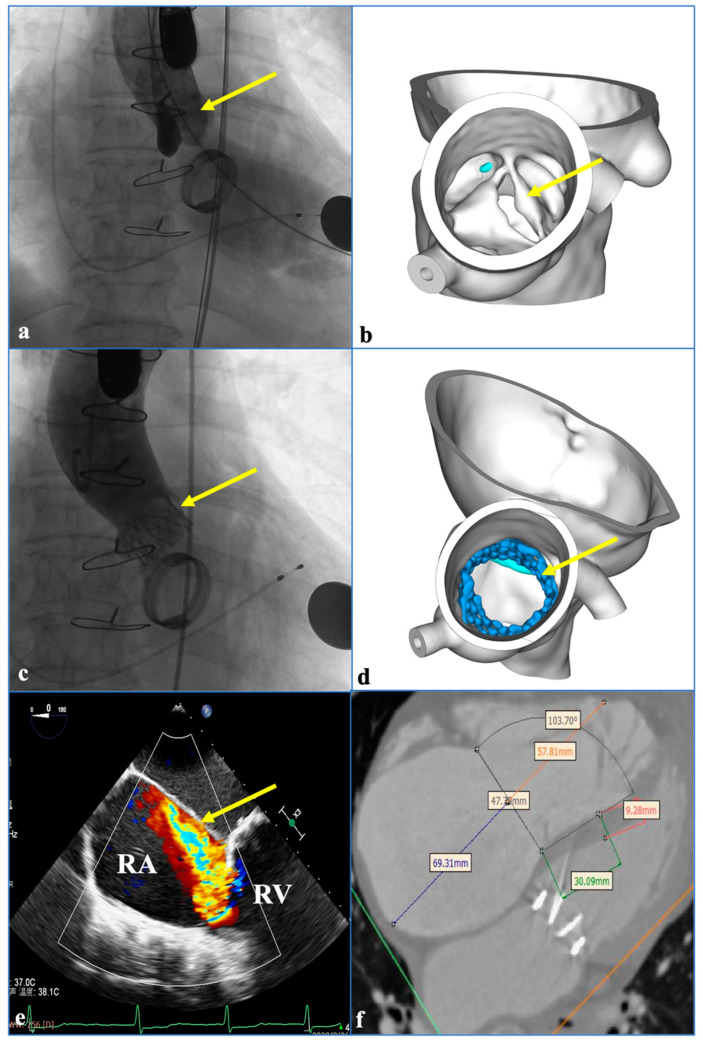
Images before transcatheter tricuspid valve replacement. (a) Digital subtraction angiography image before transcatheter aortic valve replacement (TAVR) in 2021; the arrow indicates the patient’s aortic stenosis. (b) Three-dimensional reconstruction before TAVR; the arrow points to the patient’s aortic stenosis. (c) Digital subtraction angiography shows that the aortic valve stent is fixed securely in place; the arrow points to the stent. (d) Three-dimensional reconstruction after TAVR; the arrow points to the stent. (e) The yellow arrow points to the massive regurgitation in TV. Chinese words in Figure 2e are temperature. (f) The assessment of the computed tomography angiography images before TTVR. RA: right atrium; RV: right ventricle.
